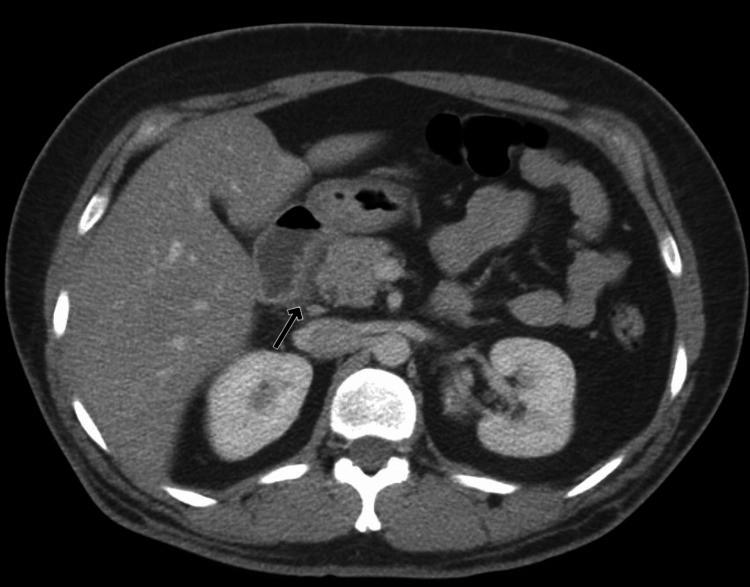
Axial CT abdomen with groove pancreatitis Arrow pointing towards fluid interdigitating between the descending portion of the duodenum and pancreas.
